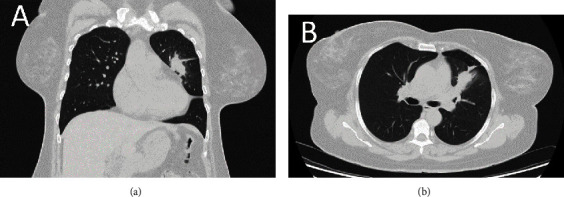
Computed tomography scan showing a mass in the upper part of the left lung: (a) coronal view and (b) sagittal view.
